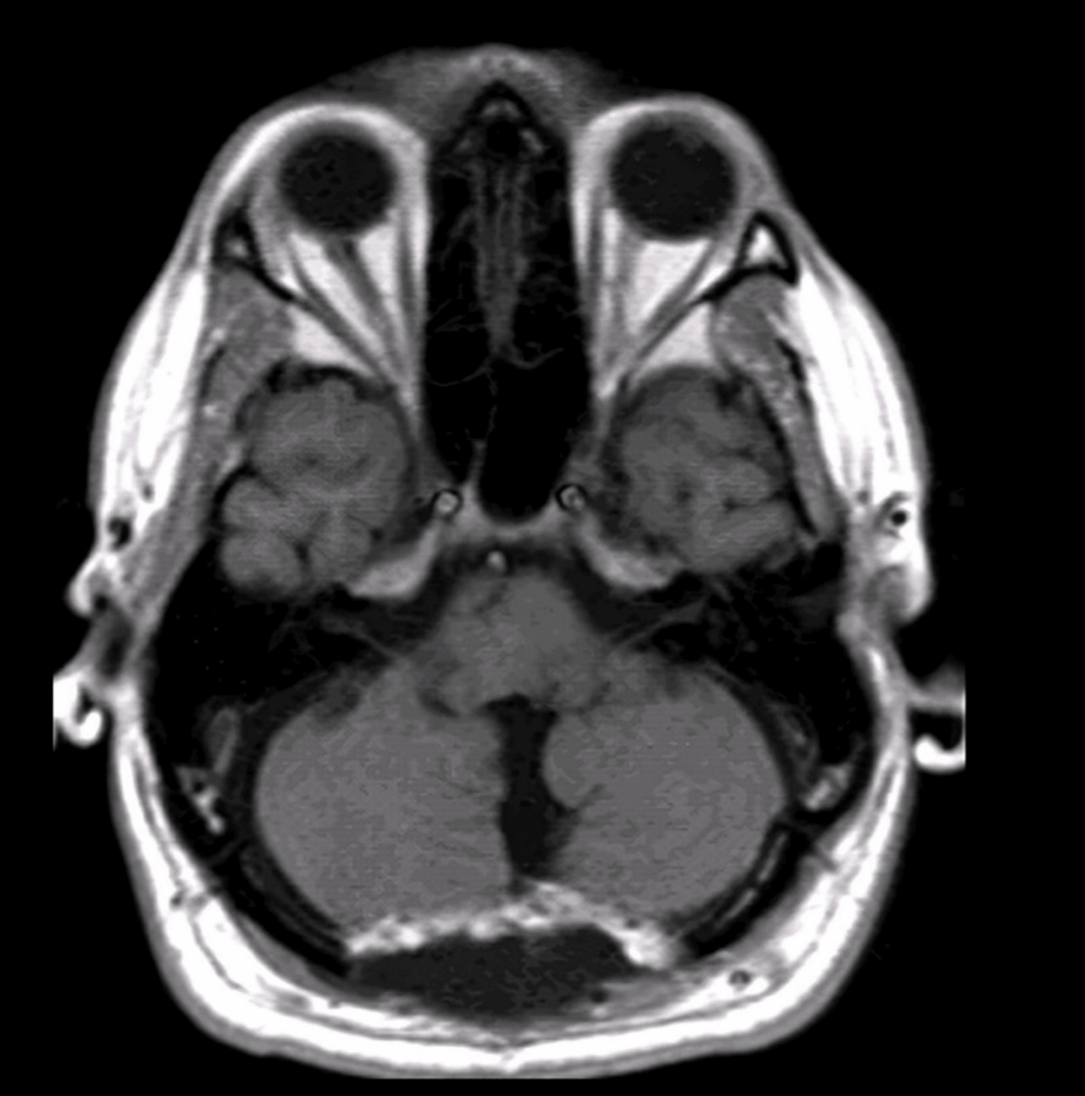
Six-month postoperative axial MRI showing postoperative changes in the posterior fossa without evidence of recurrence.
